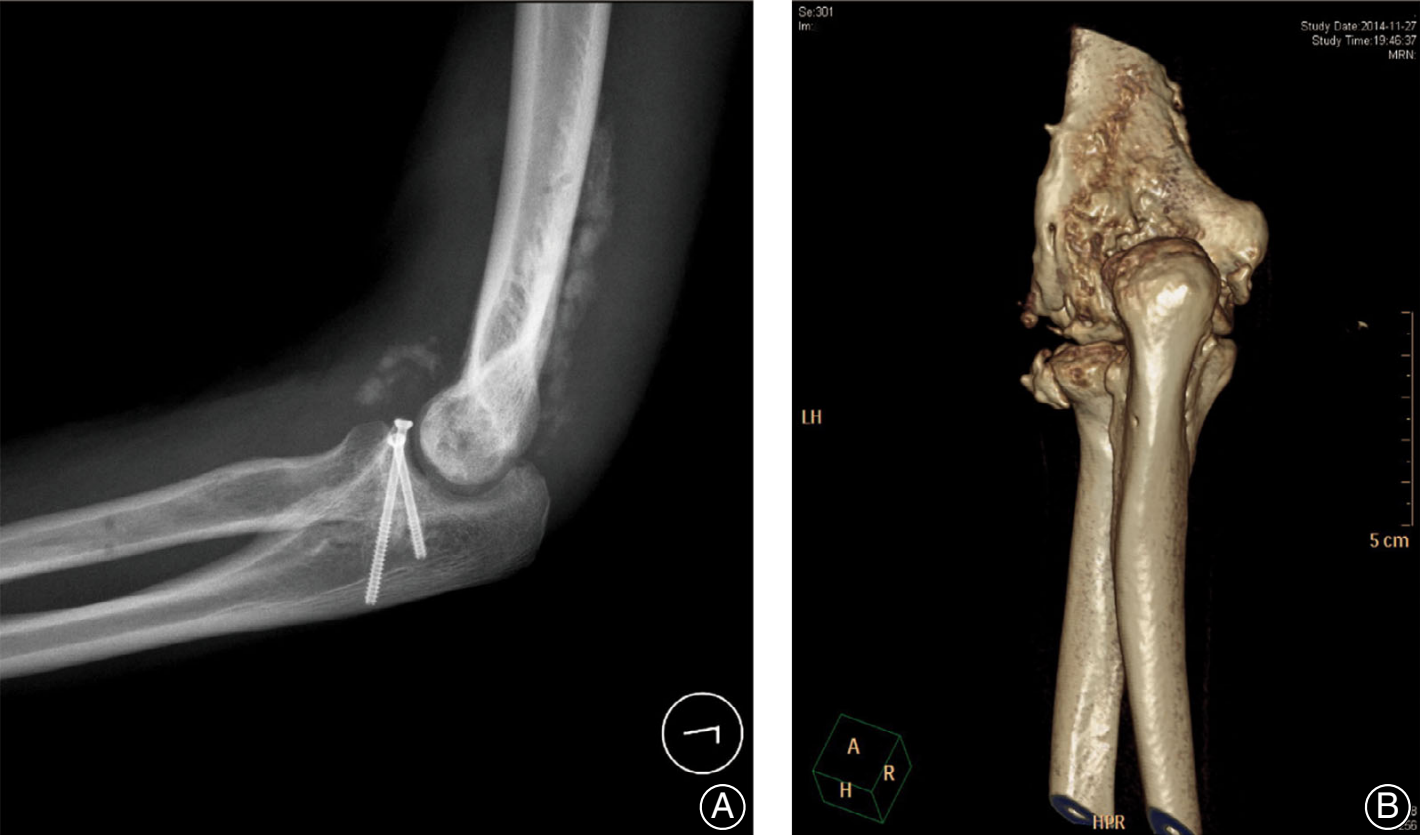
(A) Postoperative X‐ray shows heterotopic ossification (HO) formation at 1‐month follow‐up. (B) CT scan shows HO 6 months after surgery.
